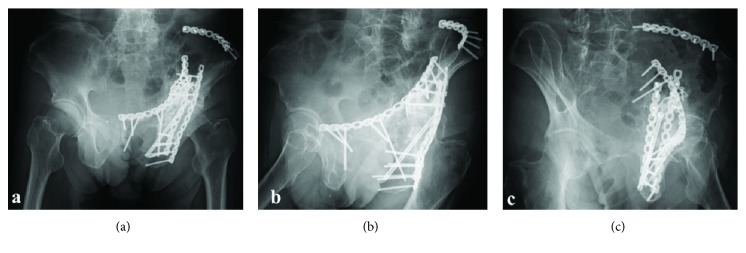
(a) Anteroposterior view and (b, c) Judet views of radiographs at the final follow-up, 18 months postoperatively.
